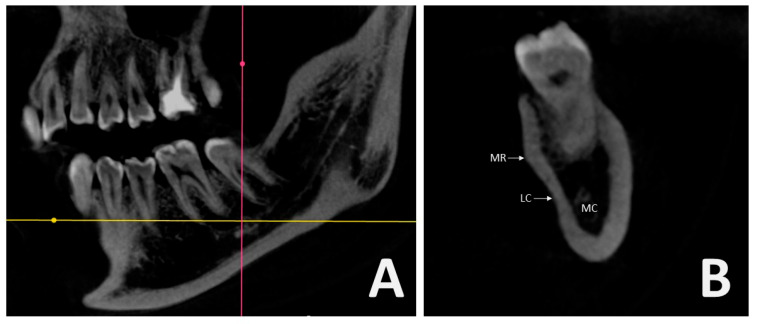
Sagittal and cross-sectional hemimandible on CBCT: (A) Point between the second molar and first molar tooth root in the sagittal image; (B) Cross-sectional images showing mylohyoid ridge (MR), lingual concavity (LC), mandibular canal (MC).
